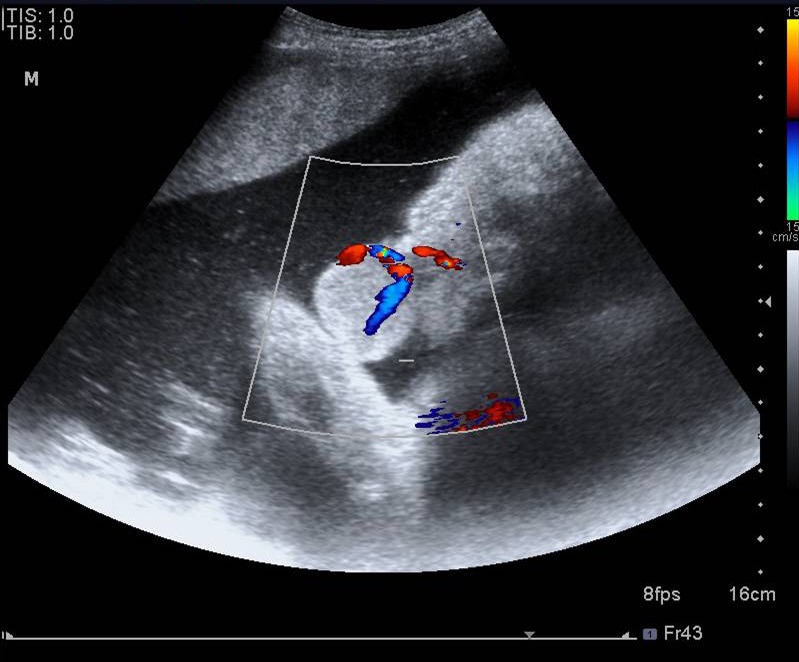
Ultrasound evaluation with color Doppler, showing a mass protruding from the mouth with a branching pattern of the feeder vessels.
